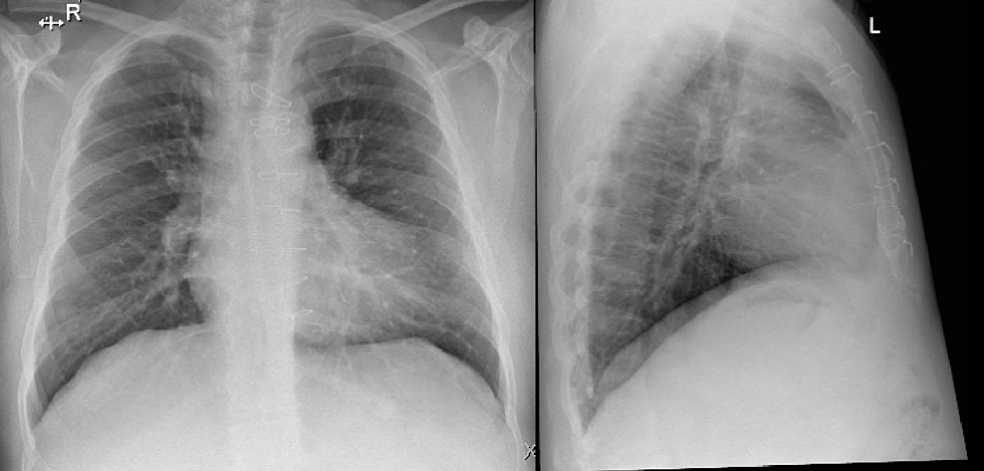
Chest x-ray of the patient at presentation
